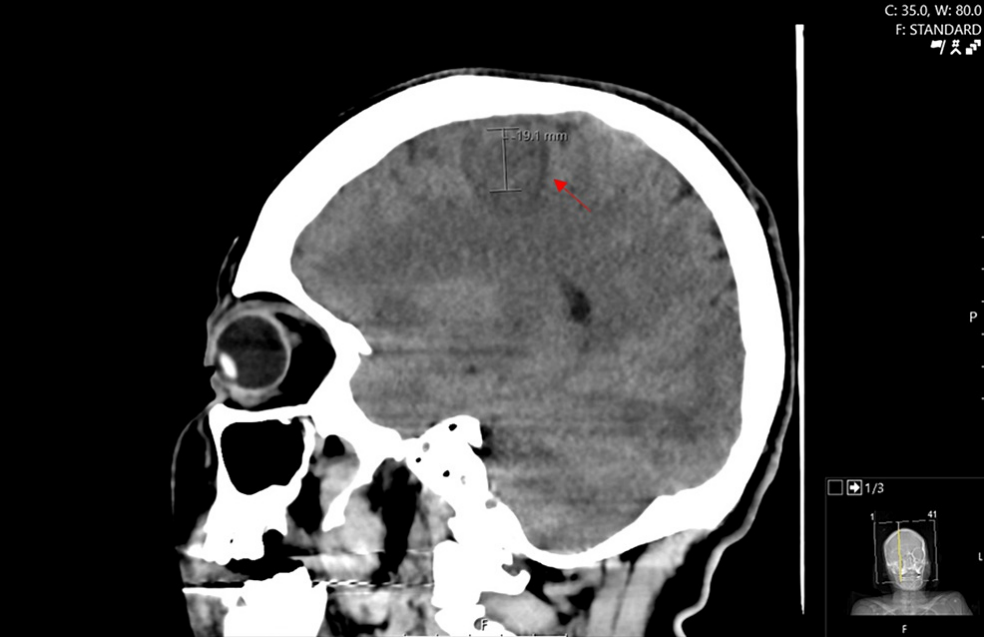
CT scan sagittal plane at admission showing a focal region of hypodensity in the right frontal lobe with surrounding hyperdensity CT: computed tomography.
